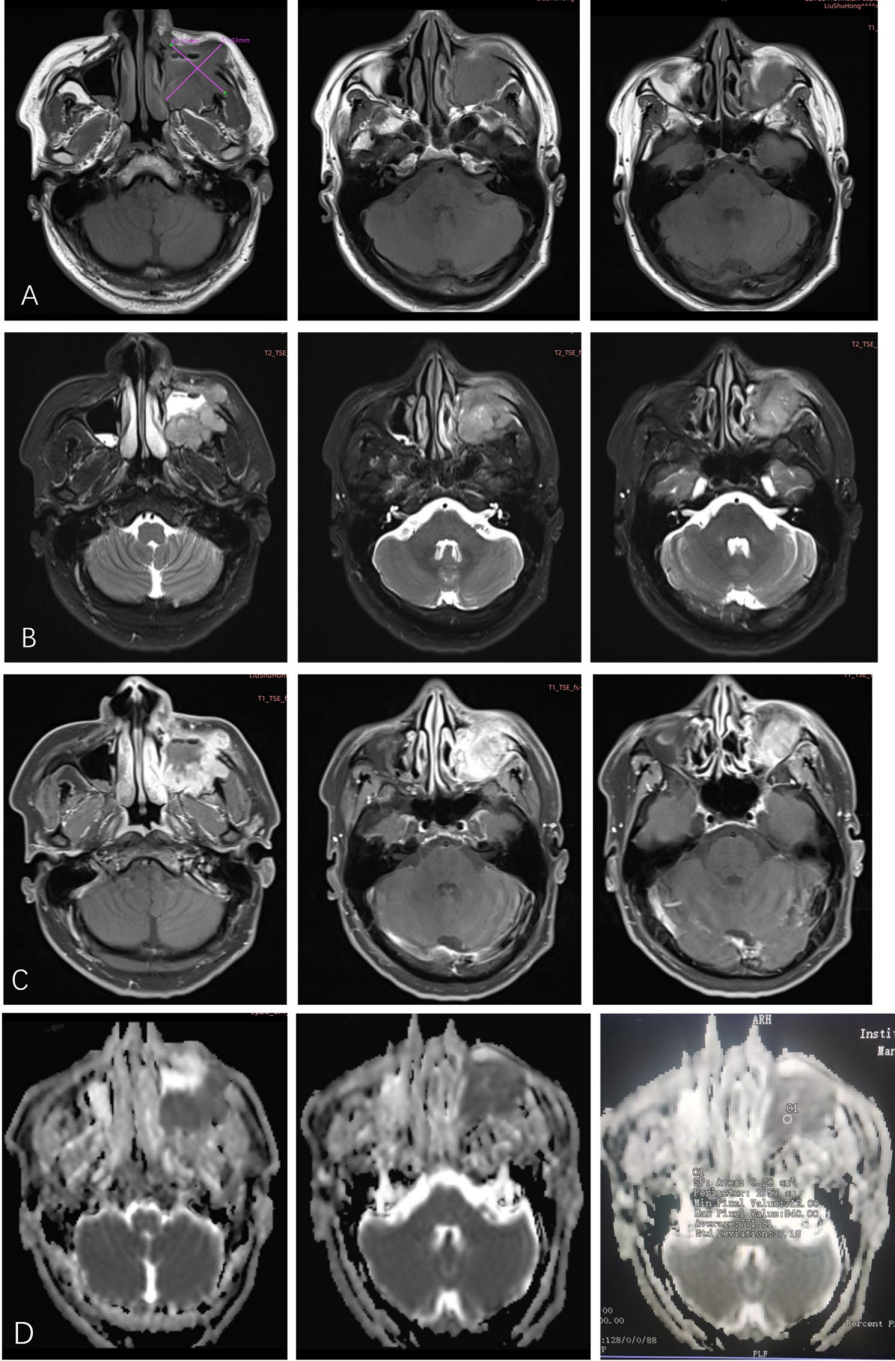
Enhanced Maxillofacial MRI from March 8, 2021, demonstrating local recurrence. A T1-weighted image showing an irregular hypointense mass (5.2 cm × 4.7 cm) in the left maxilla. B T2-weighted image showing soft tissue swelling with indistinct margins blurring the boundary with the ethmoid sinus. C Contrast-enhanced T1-weighted image showing marked heterogeneous enhancement filling the sinus and infiltrating the subcutaneous fat. D ADC showing low signal intensity within the mass, confirming restricted diffusion characteristic of tumor recurrence
